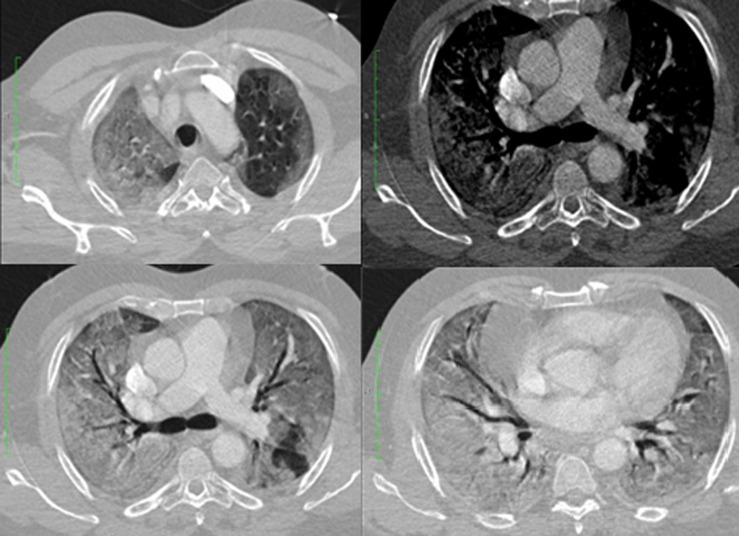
computed tomography images of case 3 on the 3^rd^ day of onset of symptoms showing bilateral lung consolidation (white lung) with a pan-lobar affectation and air-bronchograms; peripheral ground-glass opacification in the left upper lobe; no evidence of pulmonary embolism was seen
